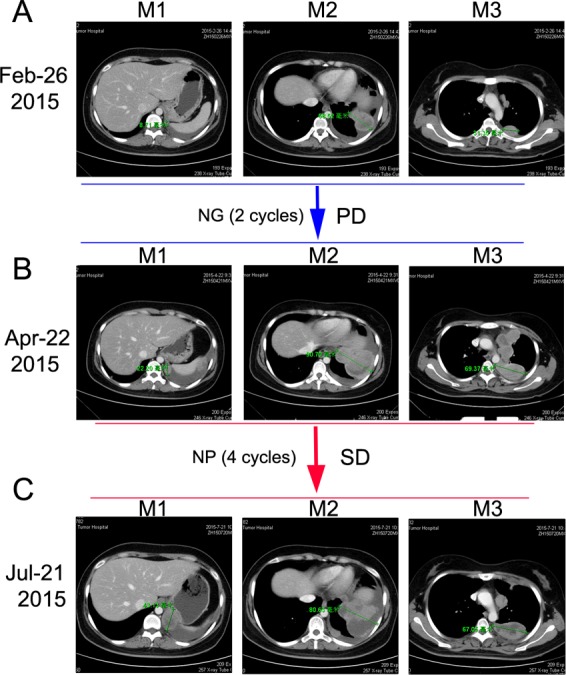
Chest CT scans documenting responses to nedaplatin and gemcitabine (NG) and nedaplatin and albumin-bound paclitaxel (NP) chemotherapies A. Baseline CT image before NG treatment. B. After two cycles of NG treatment, CT image showing a PD. C. After 4 cycles of NP treatment, CT image showing a SD.
